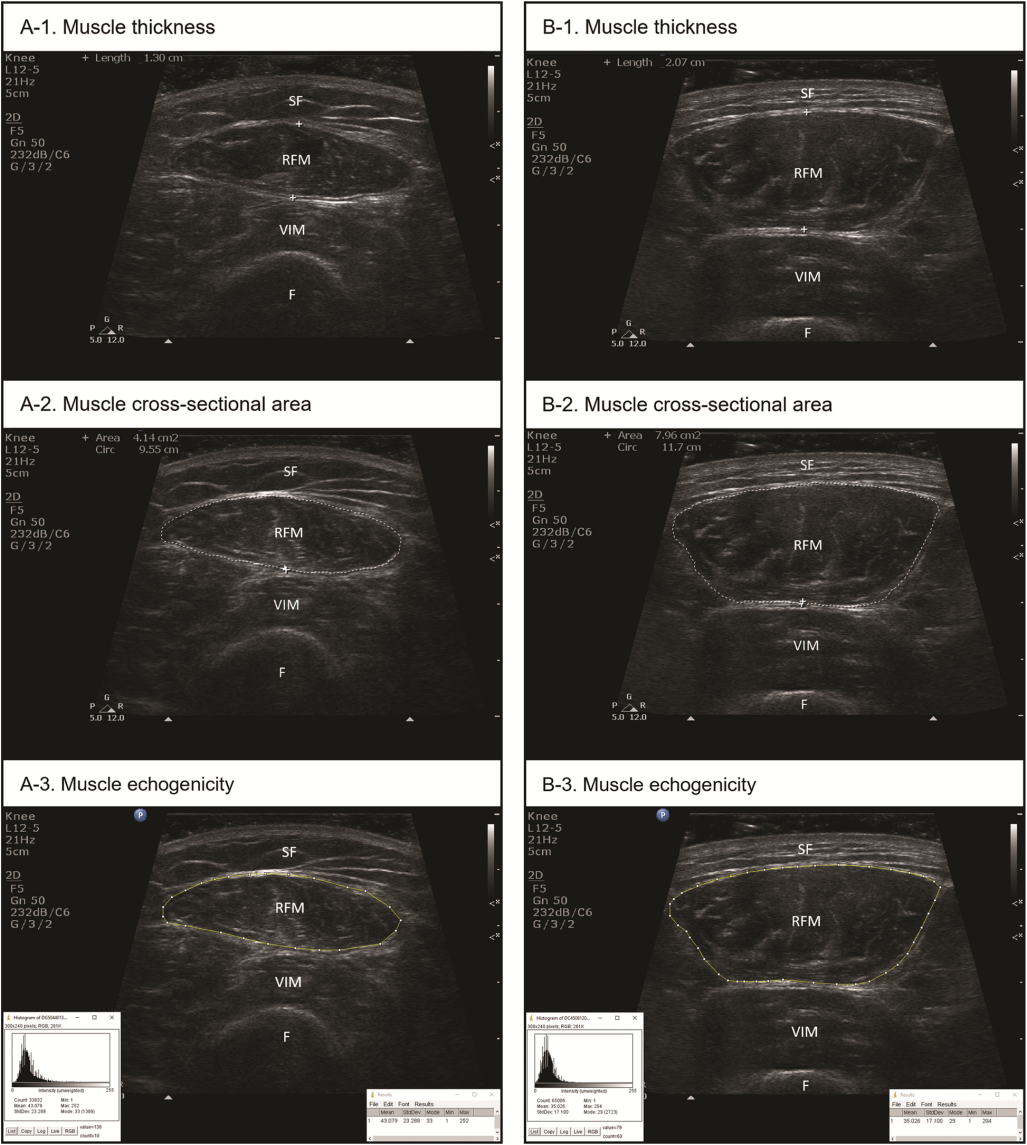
Typical transverse ultrasound images of (A) frail and (B) non-frail participants. (A-1) muscle thickness, (A-2) cross-sectional area and (A-3) echogenicity of rectus femoris muscle of a 62-year-old frail male participant. (B-1) muscle thickness, (B-2) cross-sectional area and (B-3) echogenicity of rectus femoris muscle of a 61-year-old non-frail male participant. F, femur; RFM, rectus femoris muscle; SF, subcutaneous fat; VIM, vastus intermedius muscle
